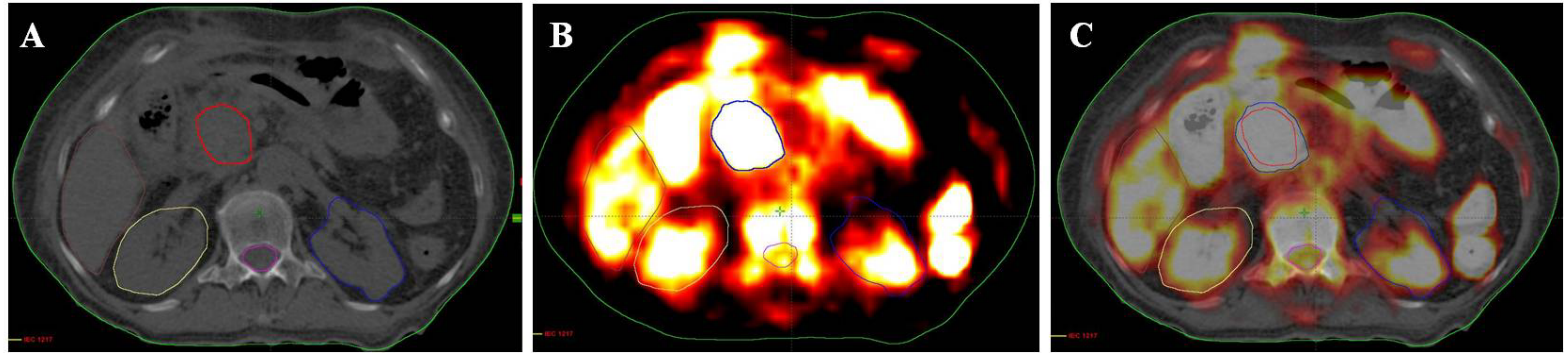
Representative image of a patient with different GTV delineations; CT (A), PET (B), and co-registered PET-CT.
